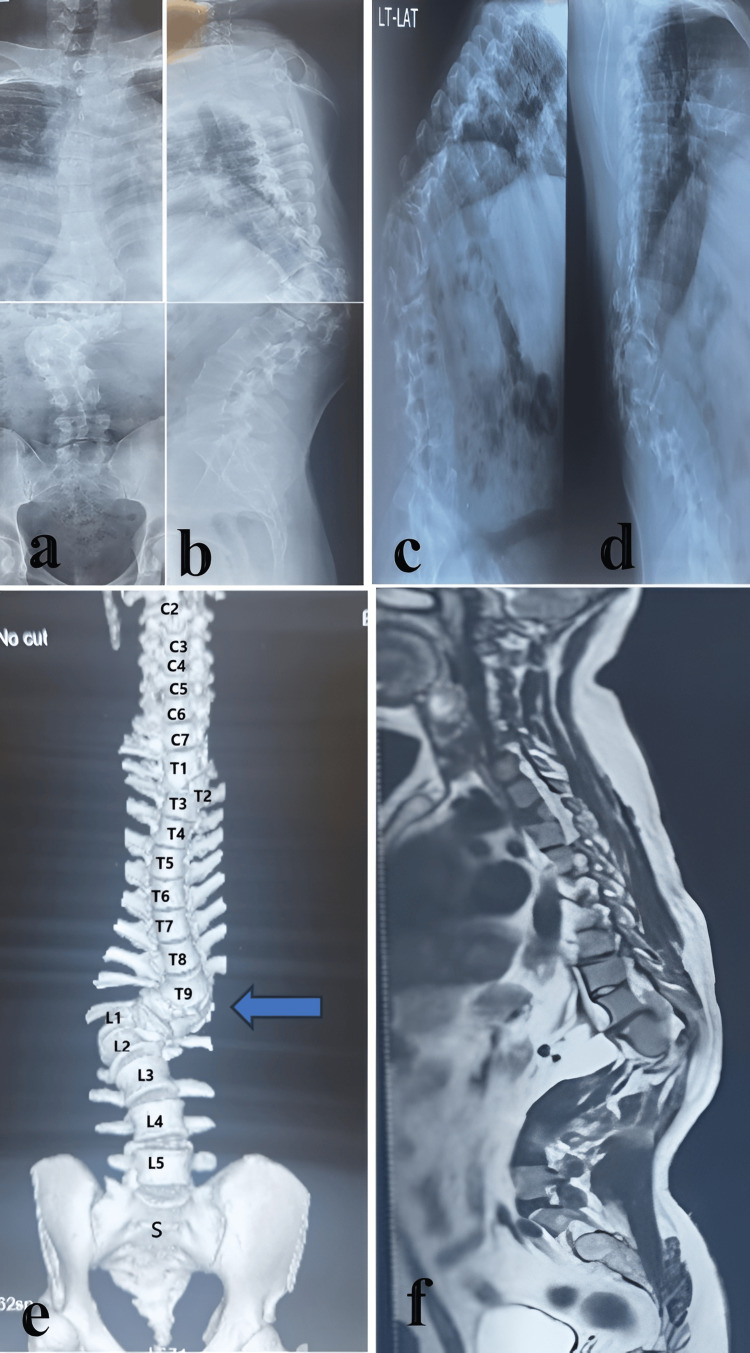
Preoperative imaging of the patient (a and b) Anteroposterior and lateral plain radiographs demonstrating spinal deformity. (c and d) Dynamic flexion and extension X-rays showing rigidity of the deformity. (e) Preoperative 3D-CT reconstruction revealing significant spinal deformity extending from below the T9 level to L1, with vertebral aplasia and absence of the left 12th rib (blue arrow). (f) Preoperative MRI showing no evidence of intraspinal anomalies or spinal cord malformations.
